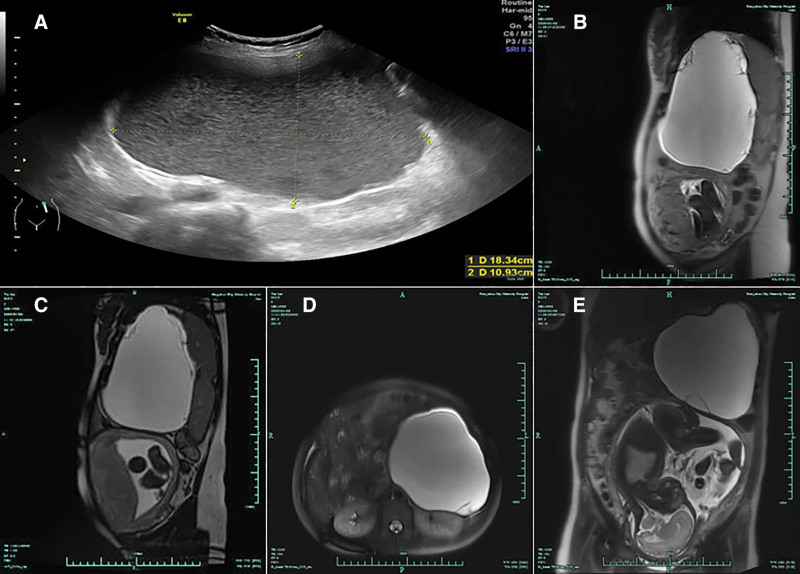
Ultrasound imaging of the PMCN. The tumor, measuring 18.34/10.93 cm, was unilocular, with a thick external wall, with no papillary projection inside (A). Magnetic resonance imaging (MRI) of the patient at 36 weeks of pregnancy. A vertical plan—both the fetus and the PMCN can be seen (B). A transverse plane of the abdomen with the tumor (C). MRI showed a 16.7/12.1/17.6 cm cystic tumor (arrow), in which the contents were hyperintense in T2-weighted imaging (D and E). PMCN = pancreas mucinous cystic neoplasm.
